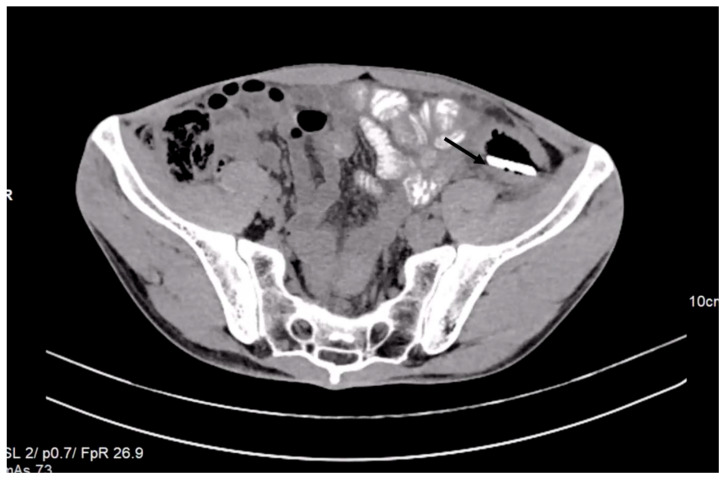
Contrast-enhanced abdominal CT revealed the presence of a foreign object in the left iliac fossa.
